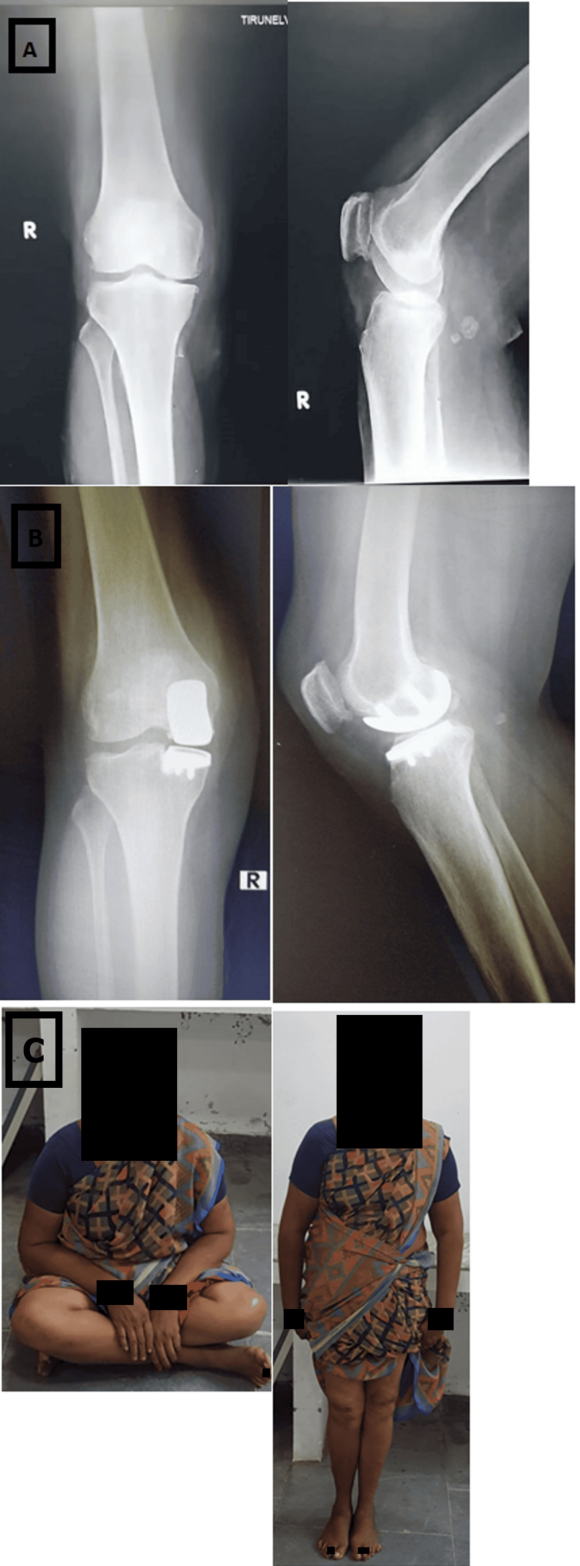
Radiographic and clinical assessment of a 58-year-old woman with severe medial knee pain (A) Pre-operative standing radiographs showing grade 3 medial osteoarthritis. The limb alignment before surgery was 9 degrees of varus. (B) Post-operative radiographs taken at a two-year follow-up. No complaint was reported by her about the knee. She could do painless stair climbing. (C) Standing radiographs show a stable and well-fixed implant and there are no signs of progression of lateral joint space narrowing.
